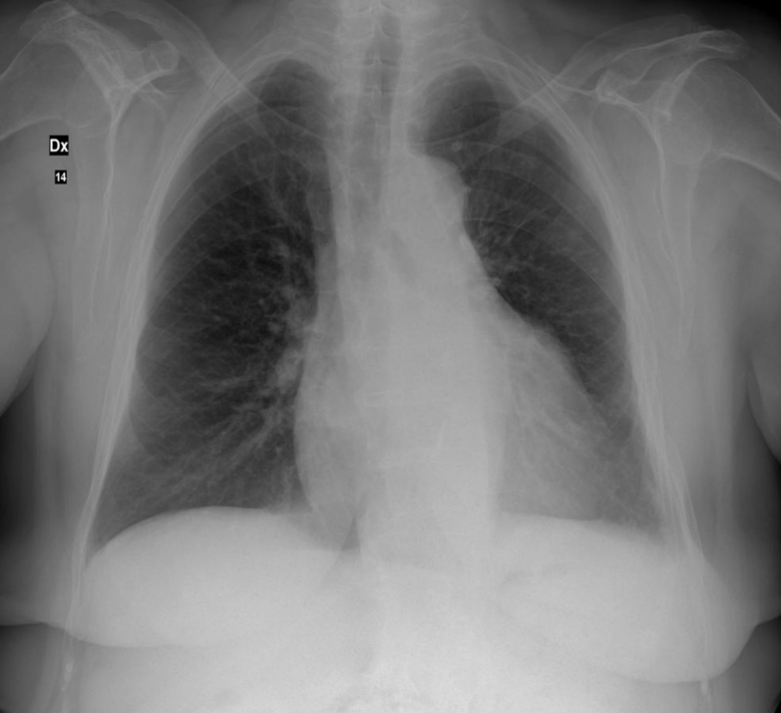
Chest antero-posterior X-ray.
